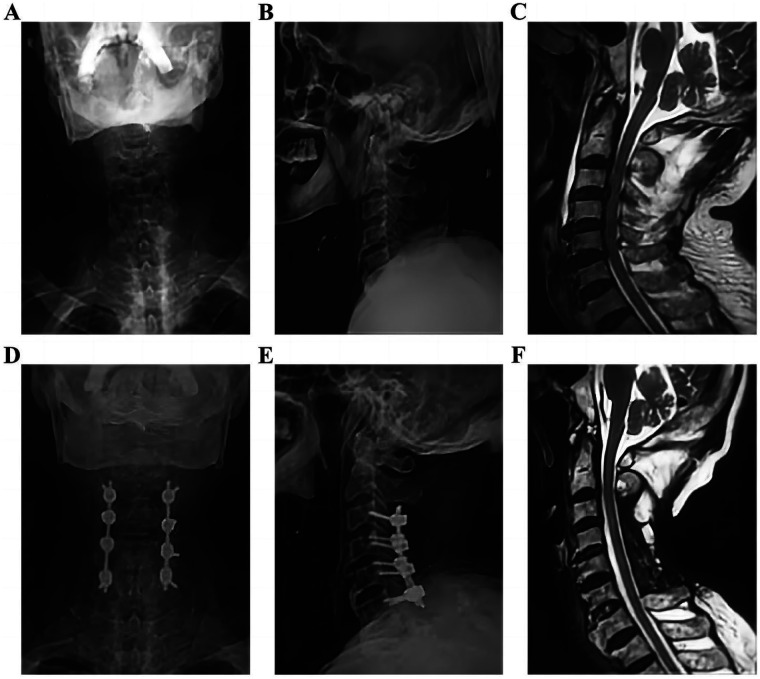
Patient 1, a 61-year-old male, presented with cervical pain and experienced bilateral upper limb numbness and weakness following an injury that occurred 3 h before admission. The diagnosis was multilevel cervical stenosis accompanied by central canal syndrome. To address this condition, the patient underwent posterior laminectomy fusion fixation. (A,B) demonstrate the presence of normal cervical lordosis without any significant narrowing in the intervertebral spaces. (C) displays multilevel cervical stenosis along with high signal intensity detected in the C3/4 segment of the cord on preoperative cervical MRI scans. (D,E) exhibit postoperative lateral and anterior-posterior x-rays of the cervical spine, indicating satisfactory positioning of internal fixation devices. (F) represents a postoperative cervical MRI scan revealing noticeable posterior displacement of the cord without substantial compression.
